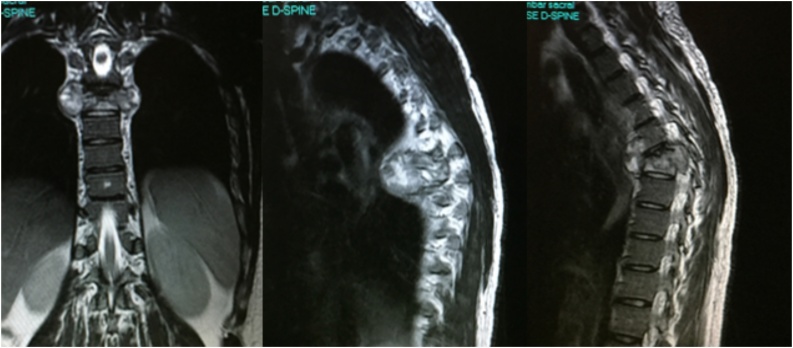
Pre-operative MRI (with contrast) scan coronal and axial images showing collapse of the D6 vertebra along with large pre and paravertebral abscess collection.
